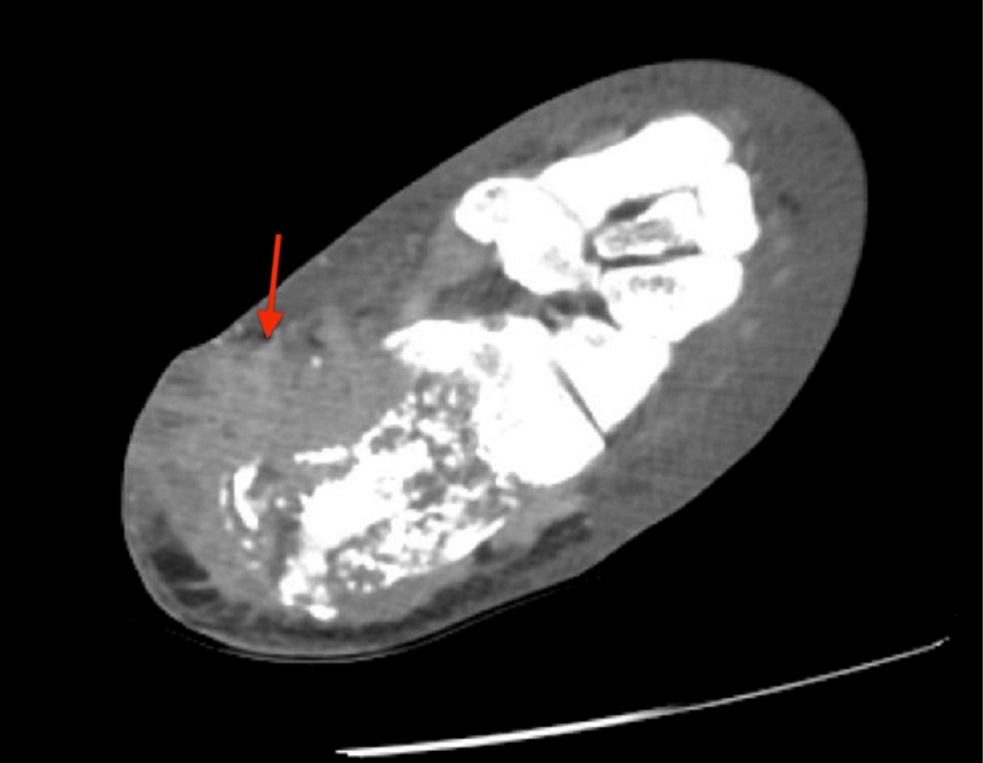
CT of the left leg with contrast showing diffuse subcutaneous fat stranding. Fluid collection within the distal Achilles tendon measuring 1.5 × 1.5 × 2.4 cm. Peripheral fluid collection of the posterior medial heel region within the subcutaneous fat measuring 4.0 × 1.7 × 2.3 cm (red arrow). CT: computed tomography
